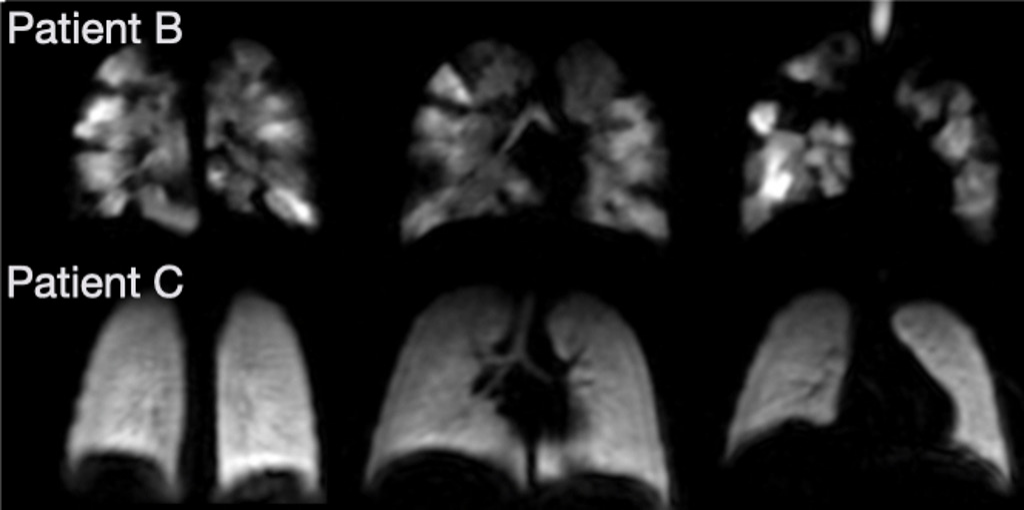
Patients B and C: Two patients with a clinical diagnosis of Fanconi Anaemia who had previously received a bone-marrow transplant. Both patients presented with similar symptoms of shortness of breath on exertion. Due to the underlying diagnosis and ionising radiation risk, the clinical team were reluctant to request a clinical CT. Both patients reported respiratory symptoms. Patient B (5 years) was unable to perform spirometry with adequate technique. Patient C had normal spirometry and TL_CO_. These patients exhibited quite different patterns of underlying ventilation; Patient B had significant ventilation defects present throughout the lungs, whilst Patient C exhibited homogeneous ventilation. Patient B therefore had underlying respiratory pathology matching the documented symptoms, whilst Patient C had normal spirometry, T_LCO_ and ^129^Xe ventilation MRI. The MR images in these two similar cases demonstrated different outcomes and allowed bespoke management plans to be created.
